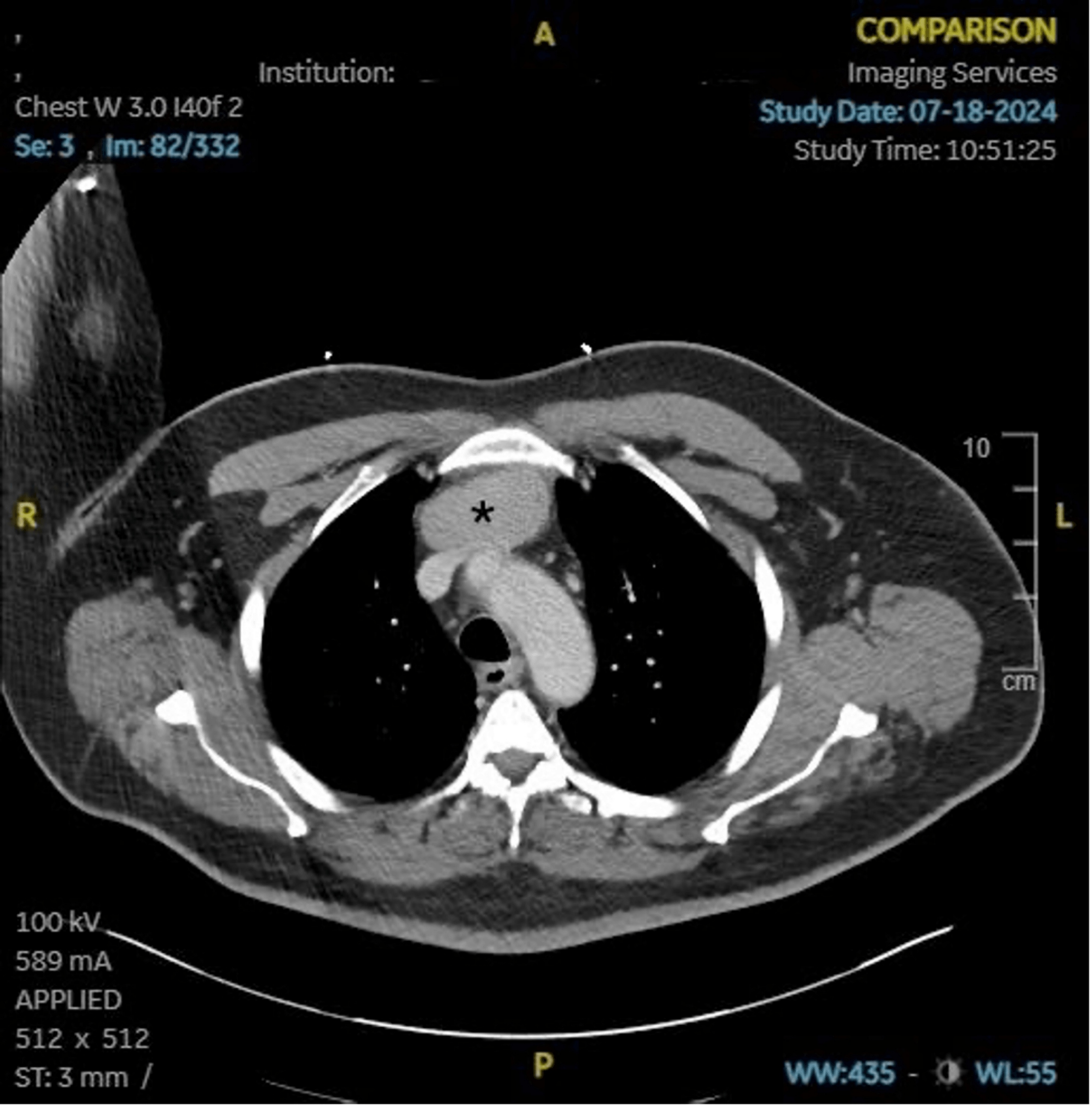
Preoperative CT chest with contrast * indicates mediastinal mass
